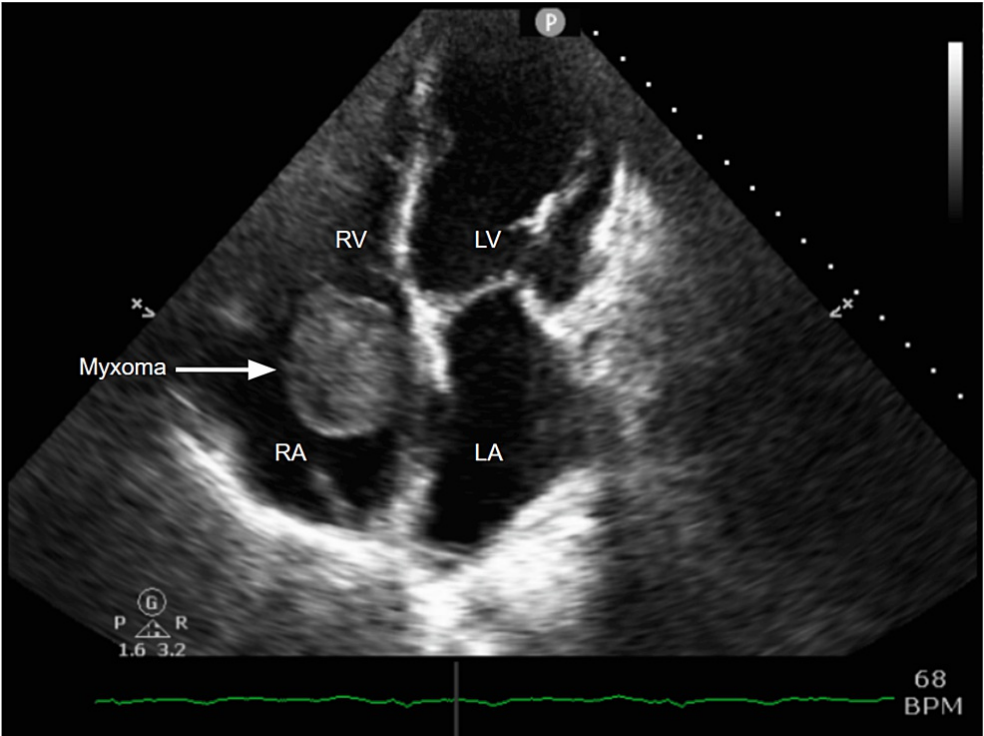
Transthoracic echocardiogram demonstrates the presence of a large right atrial mass causing right ventricular inflow obstruction RA = Right Atrium. LA = Left Atrium. RV = Right Ventricle. LV = Left Ventricle.
